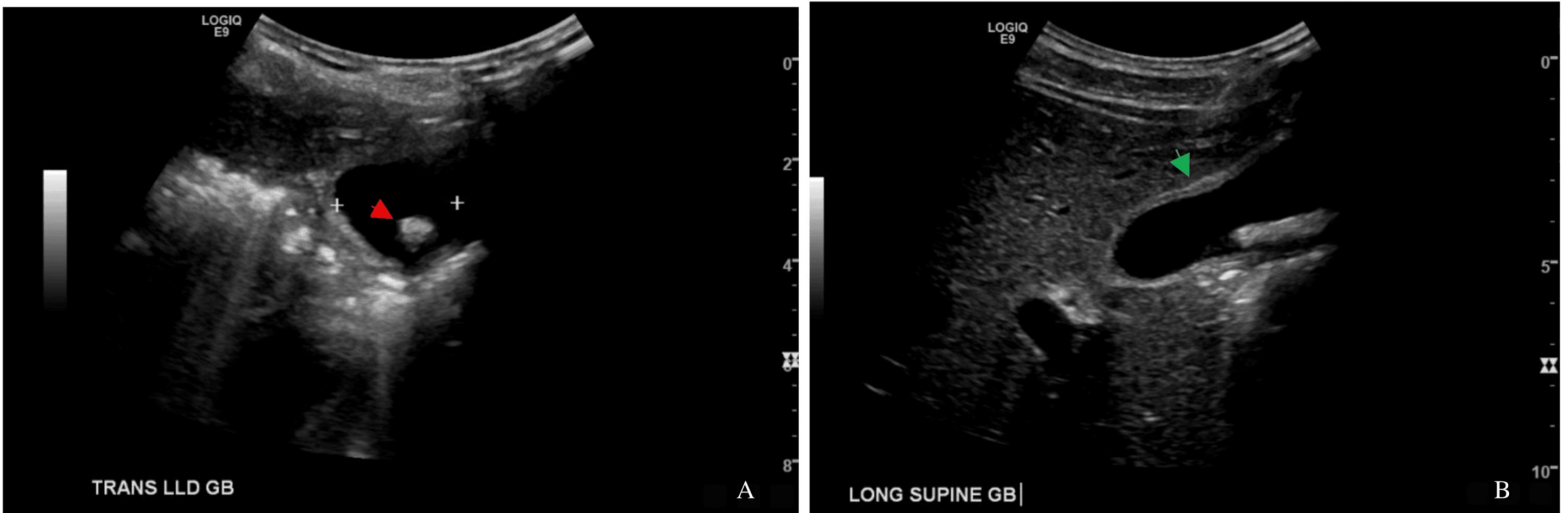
Ultrasound images of the gallbladder. A shows the gallbladder in left lateral decubitus position with a likely gallstone (red arrow); B shows the gallbladder in supine position with mild gallbladder wall thickening (green arrow).
